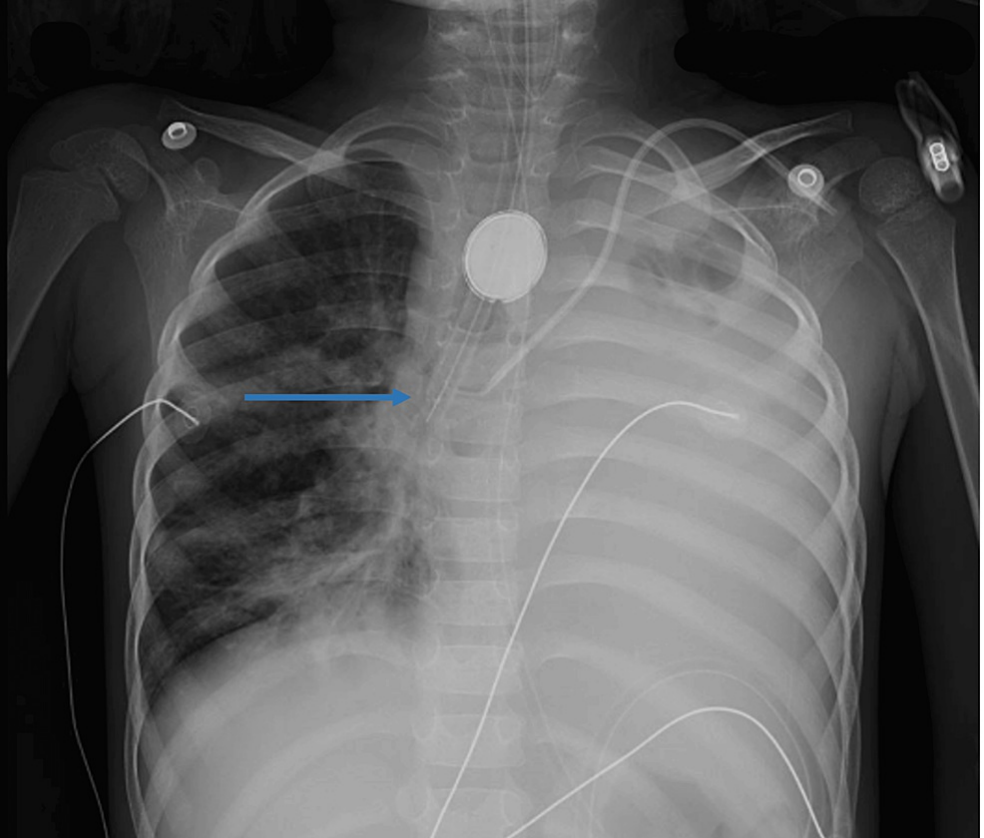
Chest X-ray after intubation Chest radiograph taken after selective right intubation. The blue arrow points to the endotracheal tube in the right bronchus.
